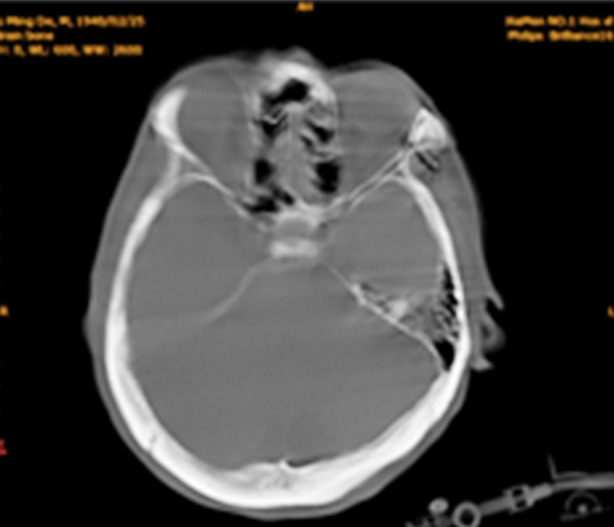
CT manifestations of CSDH
